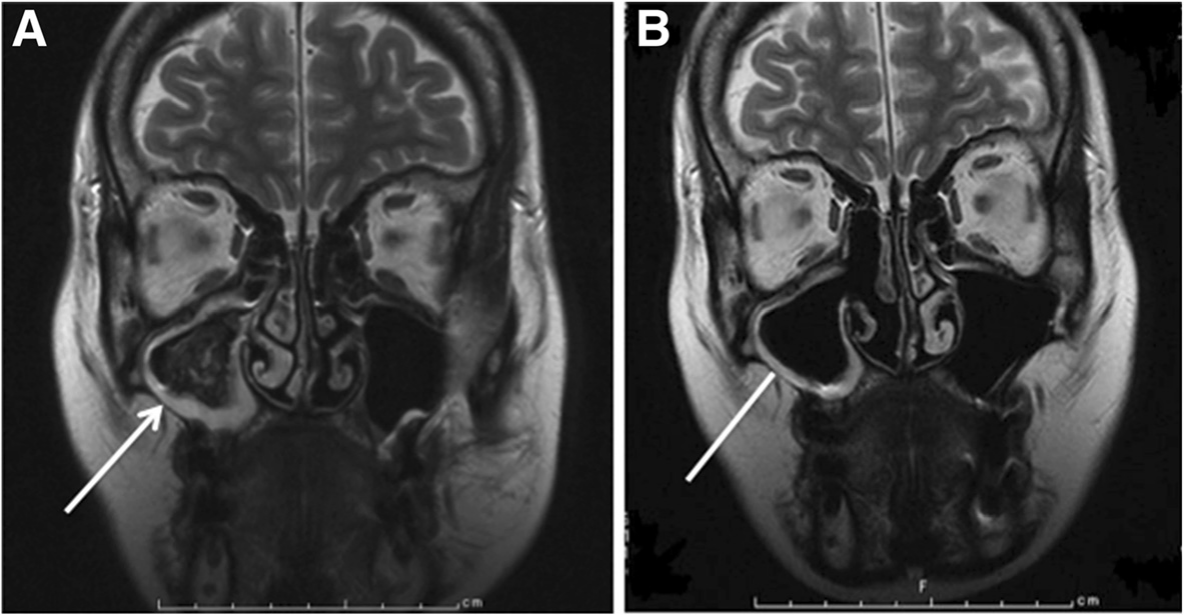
Pre- and postoperative nasal sinus magnetic resonance images are shown. Preoperative routine nasal sinus magnetic resonance image revealed diffuse inflammatory mucosal hyperplasia of the right maxillary sinus, and a mycetoma without invasive fungal sinusitis in T2-weighted image ((A), arrow). At 1 month after endoscopic treatment, diffuse inflammatory mucosal hyperplasia of the right maxillary sinus improved and mycetoma disappeared ((B), arrowhead).
